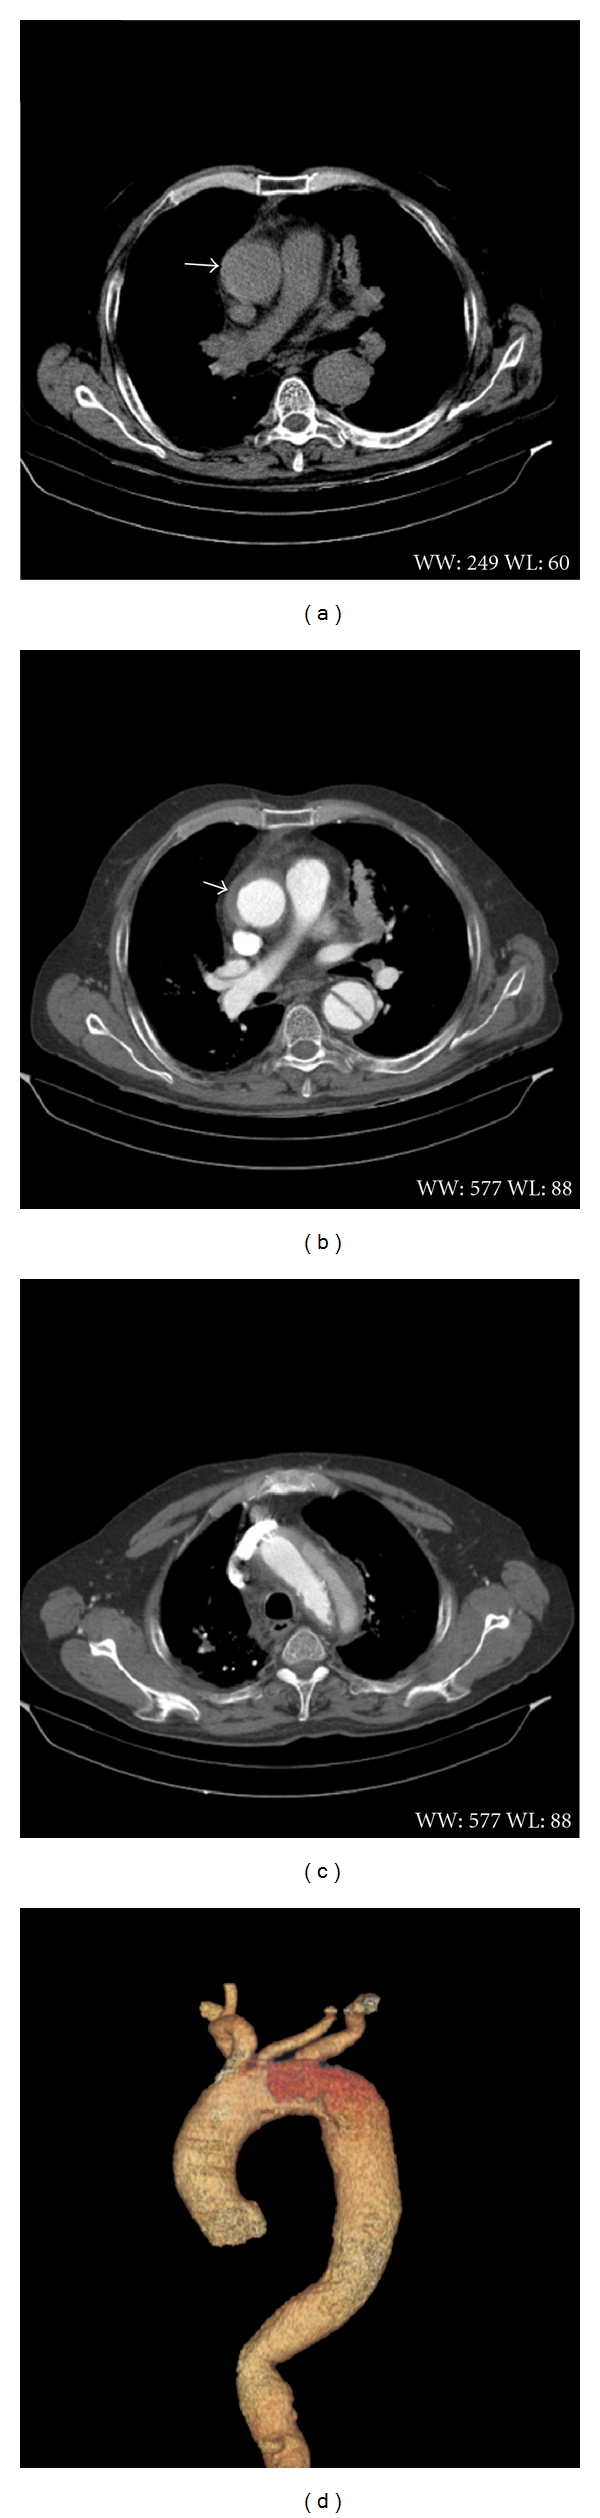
((a) and (b)) Preoperative computed tomography images demonstrating intramural hematoma in the ascending part of aorta. (c) Reveal classic dissection of the aortic arch with false and true lumen. (d) Reconstructed view.
